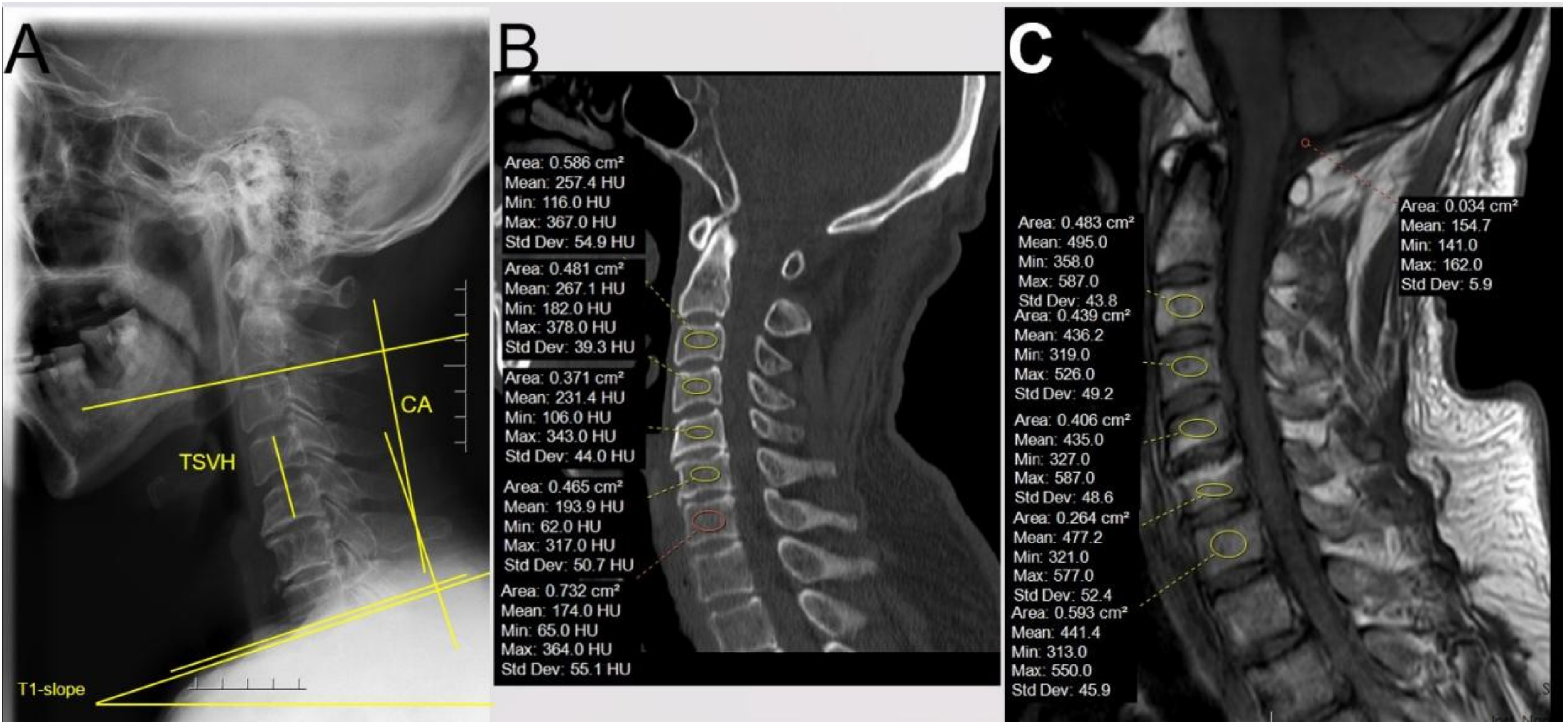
Preoperative measurement methods. (A) Different radiological parameters of x-ray. CA: the angle between the inferior endplate of C2 and the inferior endplate of C7. T1-slope: the angle between the superior endplate of T1 and the horizon. TSVH: the distance between the superior endplate of the upper vertebra and the inferior endplate of the lower vertebra of the surgical segment. (B) Measurement of Hounsfield units: the ROI was placed on cancellous, away from the endplate with more than 2 thicknesses of the endplate. (C) Measurement of C-VBQ: the ROI of the vertebra was the same as CT, and another ROI of cerebrospinal fluid was placed on the cisterna magna.
